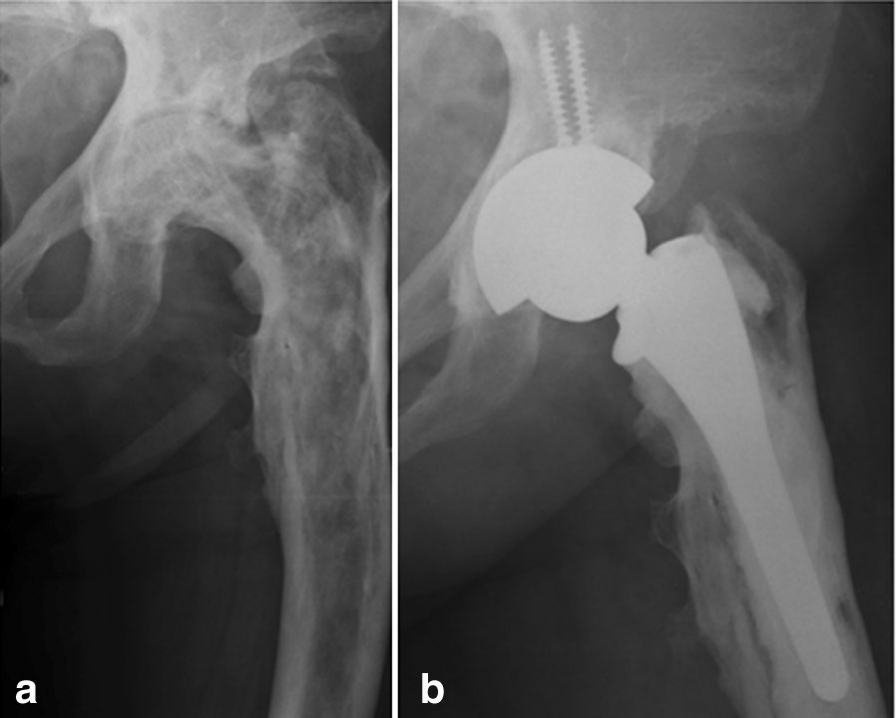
a Pre-operative anteroposterior X-ray of the hip of a woman who was 60 years old at the time of surgery; b 1-year post-operative X-ray showing wide radiolucent lines along the cement–bone interface at the stem
